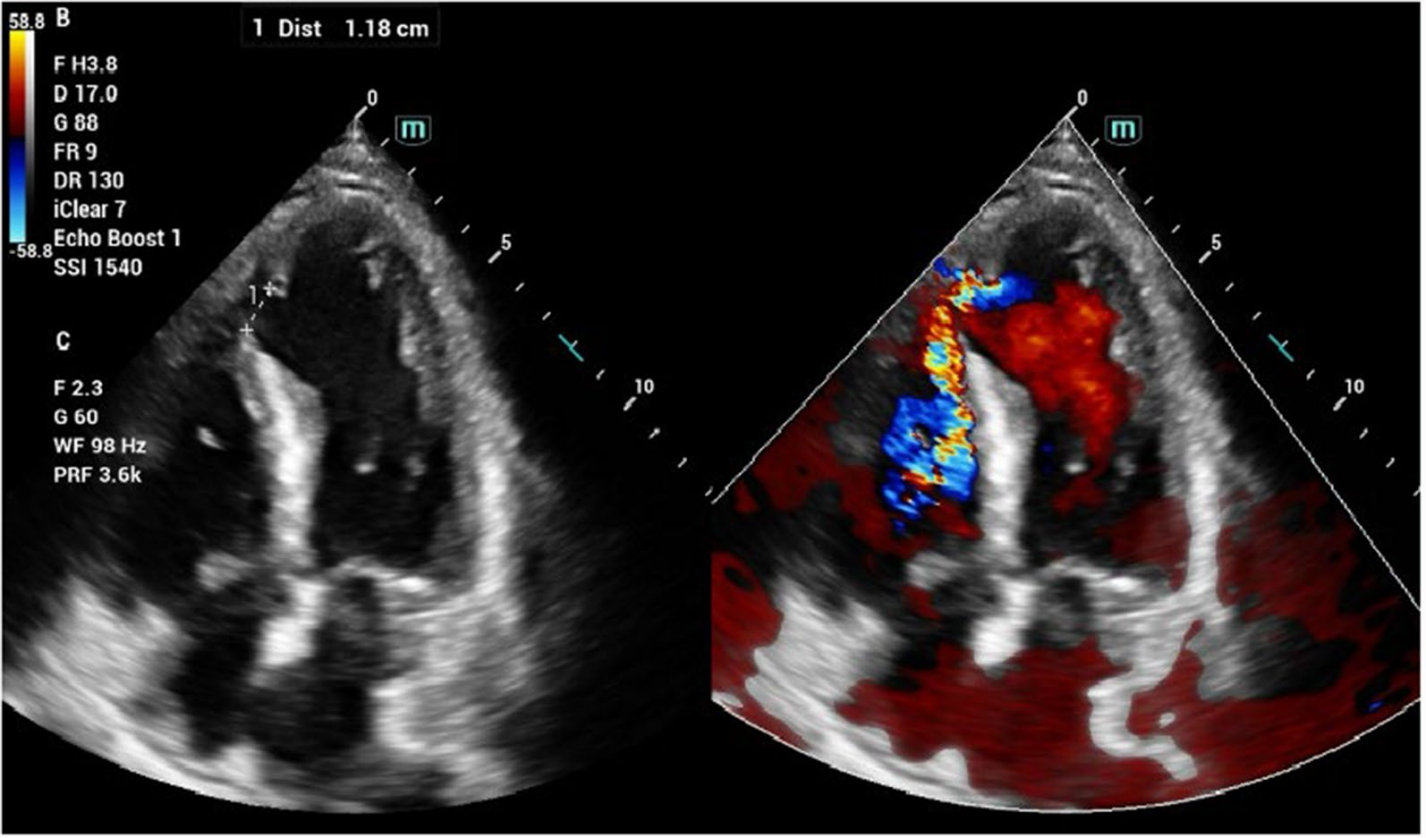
Bedside transthoracic color Doppler echocardiogram (TTE): Apical 4-chambers view shows an 11.8-mm ventricular septal rupture (VSR) located at the apical segment
